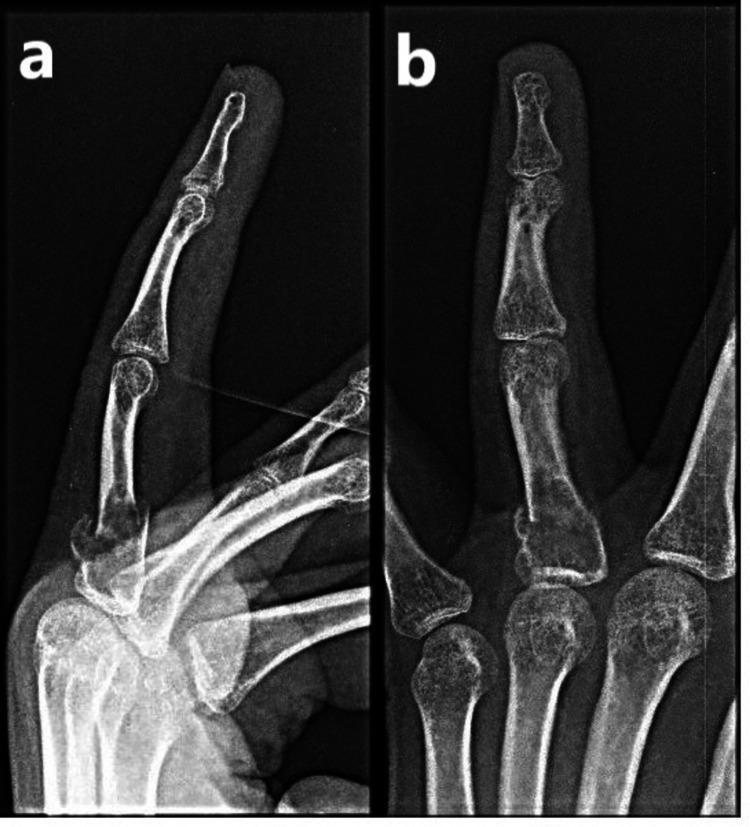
X-ray of the left ring finger. Lateral view (a) and oblique view (b) showing the fracture at the base of the proximal phalanx. Along with the fracture, there is an expansile lytic lesion seen in the metaphyseal region without cortical breach.
